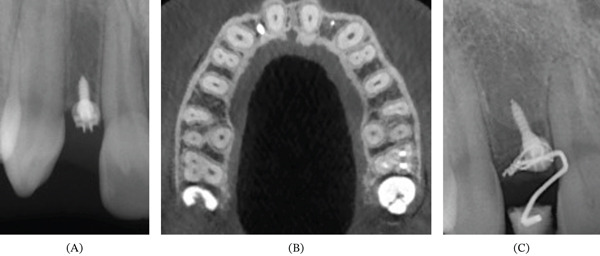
(A–C) Radiographs after insertion of the miniscrews.
